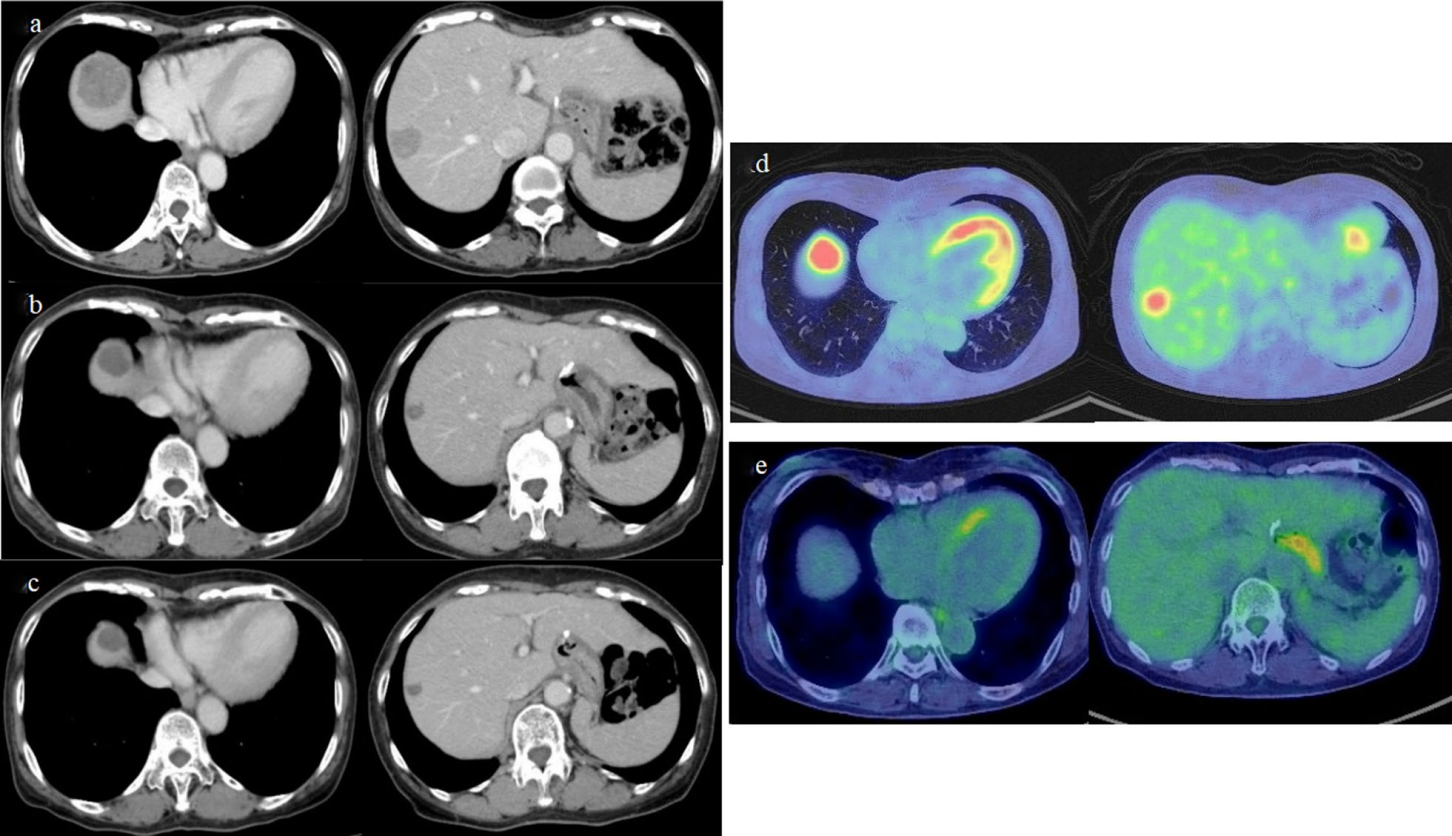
Contrast-enhanced CT and PET–CT during nivolumab treatment. a CT before nivolumab treatment. Liver metastases were 41 × 39 mm in segment 4 and 24 × 22 mm segment 8. b CT after 8 courses of nivolumab. The target lesion of liver metastasis achieved a therapeutic partial response. c CT after 18 courses of nivolumab. Liver metastases had shrunk to 24 × 22 mm in segment 4 and 11 × 9 mm in segment 8. d PET–CT at the time of recurrence and before nivolumab administration. No evidence of intrahepatic metastases other than two recurrent hepatic metastases was noted on CT. e PET–CT after 18 courses of nivolumab. No abnormal fluorodeoxyglucose (FDG) accumulation in liver metastases was shown, and no new metastases appeared
